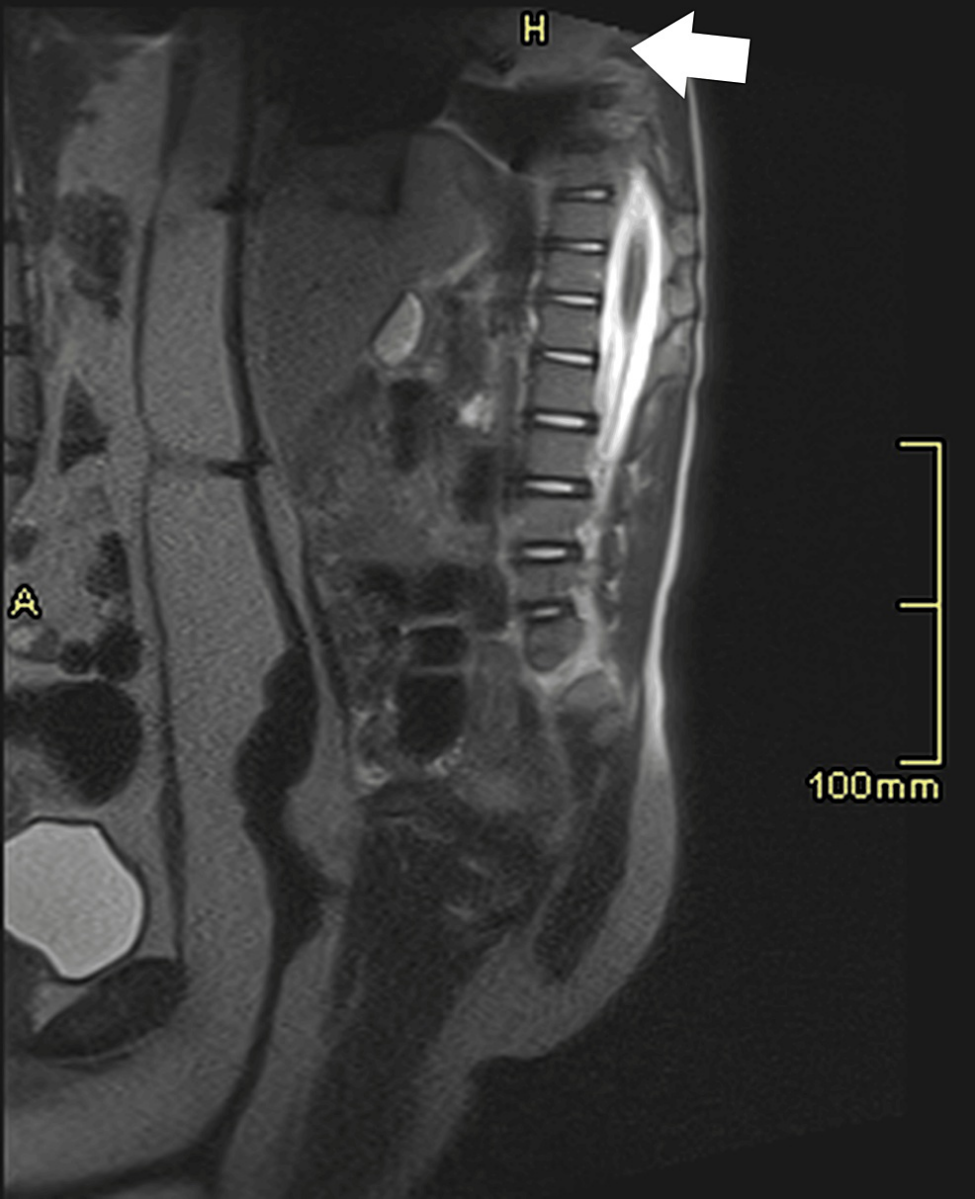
1.5 T MRI T2-weighted sagittal imaging of the same child’s abdomen and spine, with the incidental finding of a pulmonary lesion (arrow).
